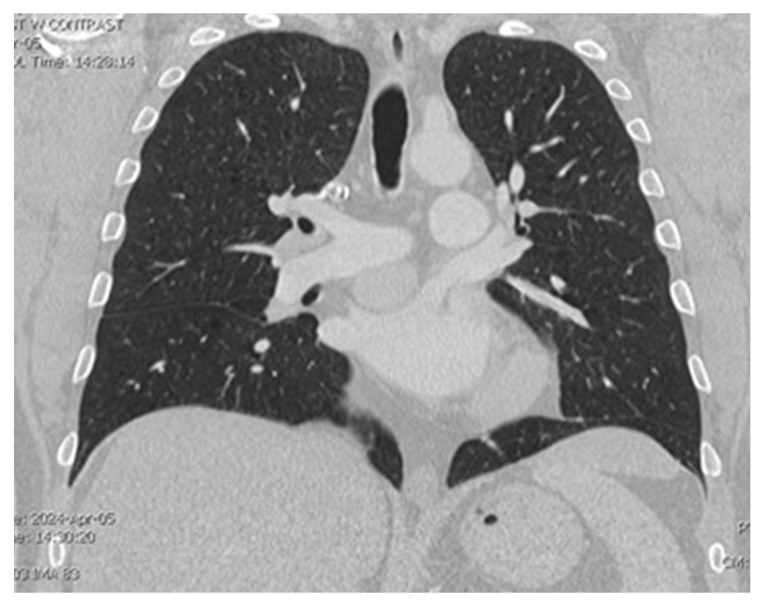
Visualization of the mass located between the pulmonary artery and left atrium on CT chest.
